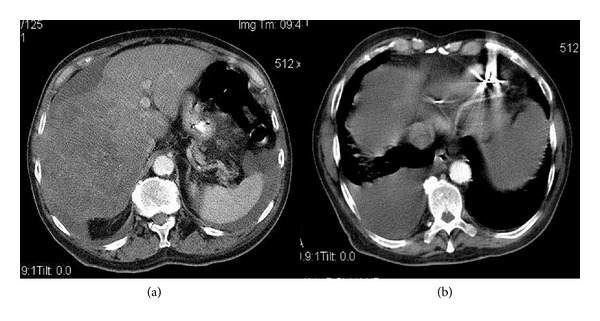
Contrast enhanced CT scan of thorax, abdomen, and pelvis.
